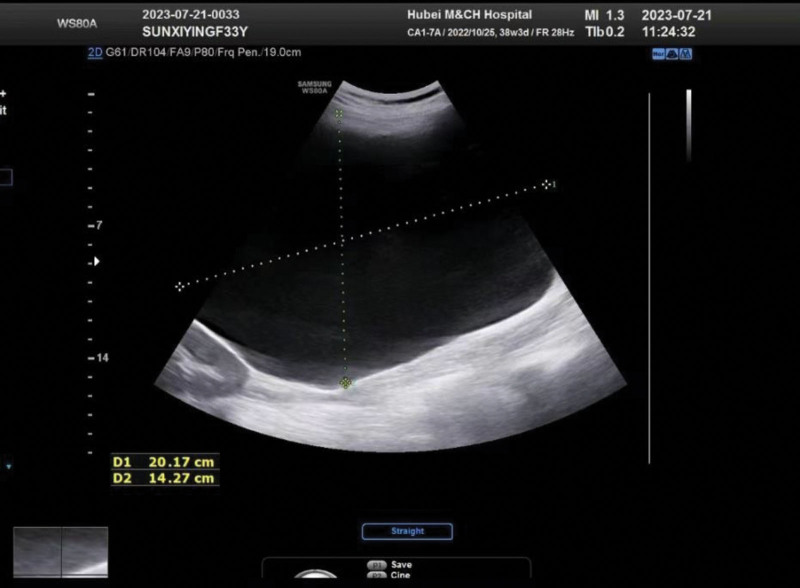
Huge retroperitoneal cystic mass shown on ultrasound.
